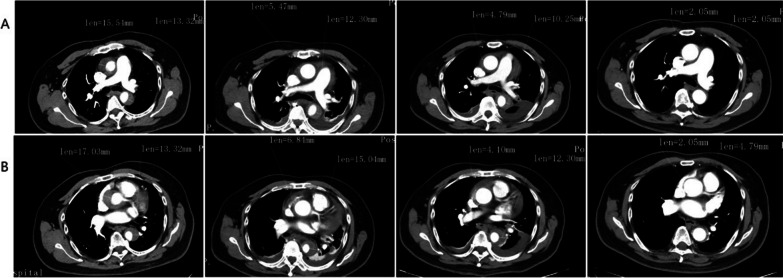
A typical case: CTA dynamic follow-up image of type A IMH patients undergoing conservative treatment. a The CTA images of pulmonary artery bifurcation plane at 1 month, 3 month, 6 month, and 12 month of the disease. b The CTA images of the left coronary artery orifice plane are 1 month, 3 month, 6 month, and 12 month
